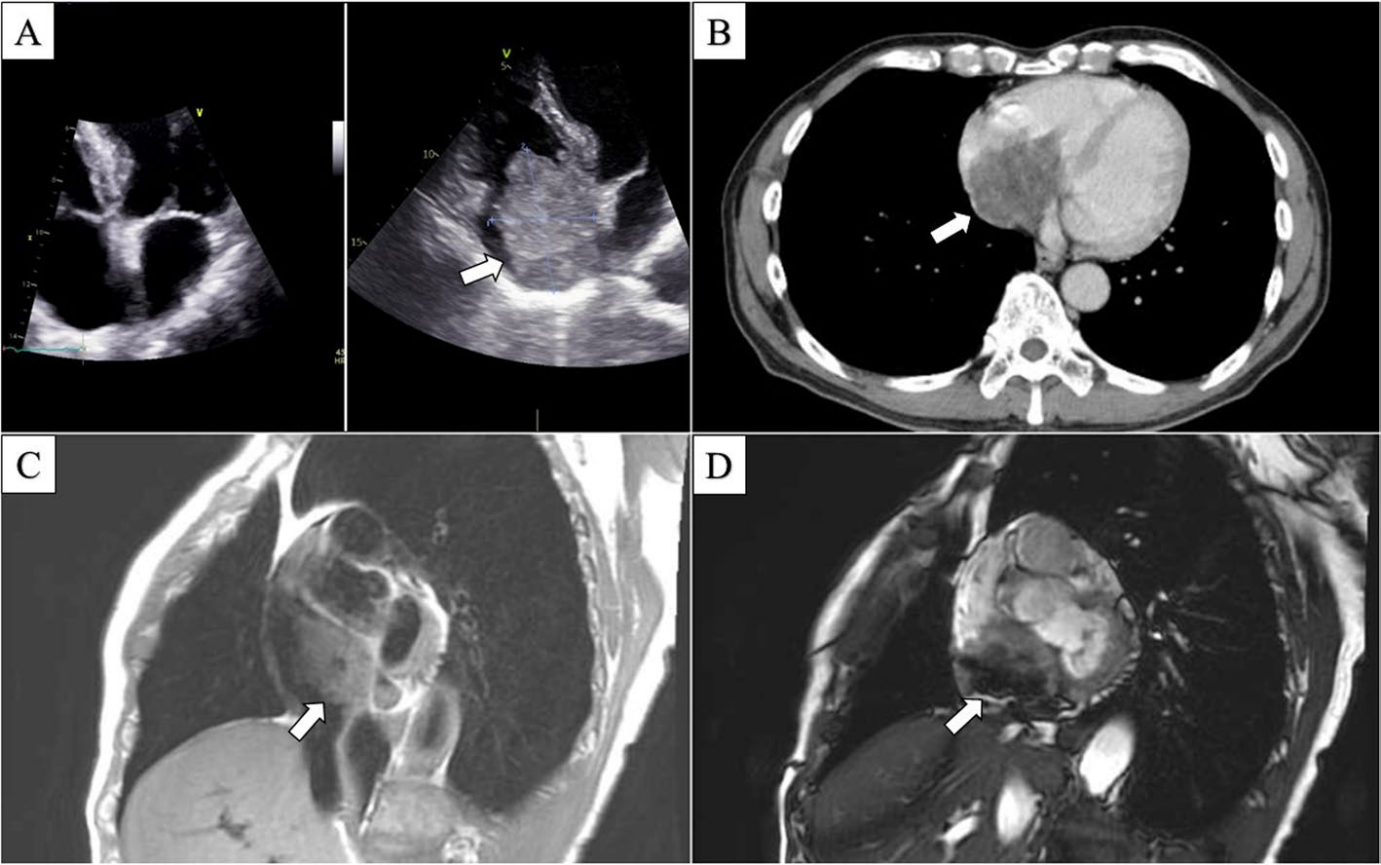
Preoperative images. A Transthoracic echocardiogram at the right after laser catheter ablation and the time of hospitalization this time. The latter demonstrates a giant mass in the right atrium (arrow). B Contrast-enhanced CT reveals the tumor has an irregular surface, heterogeneous contrast-enhanced effect, and vascular structures within. C Cardiac magnetic resonance imaging (CMR) exhibits heterogeneous high T1 signal. D CMR reveals a low-to-high T2 signal
